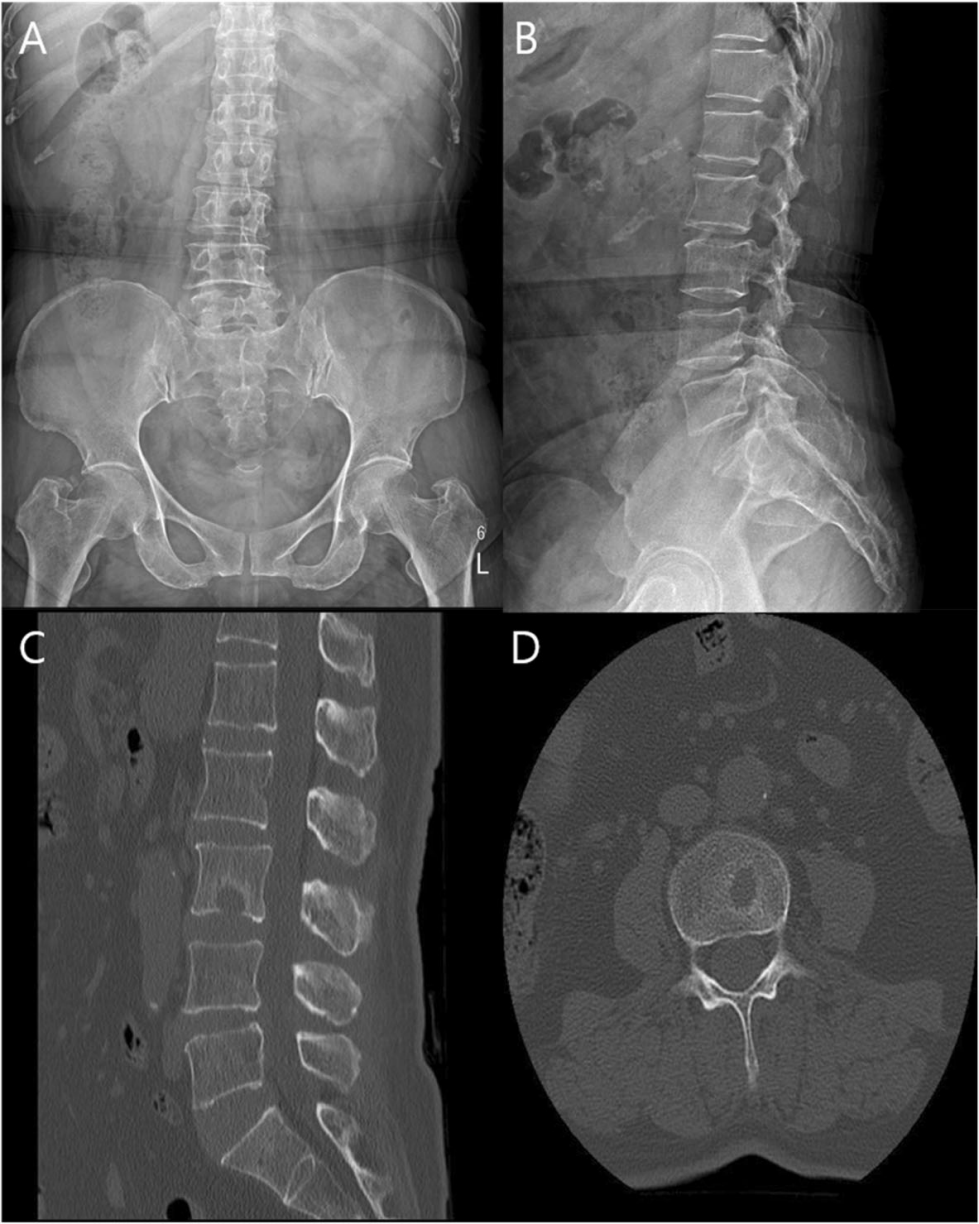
plain radiograph AP (a) and lateral view (b) showing mild degenerative scoliosis CT scan saggital (c) and axial (d) section showing schmorls node at inferior end plate of L3 vertebra with end plate defect
